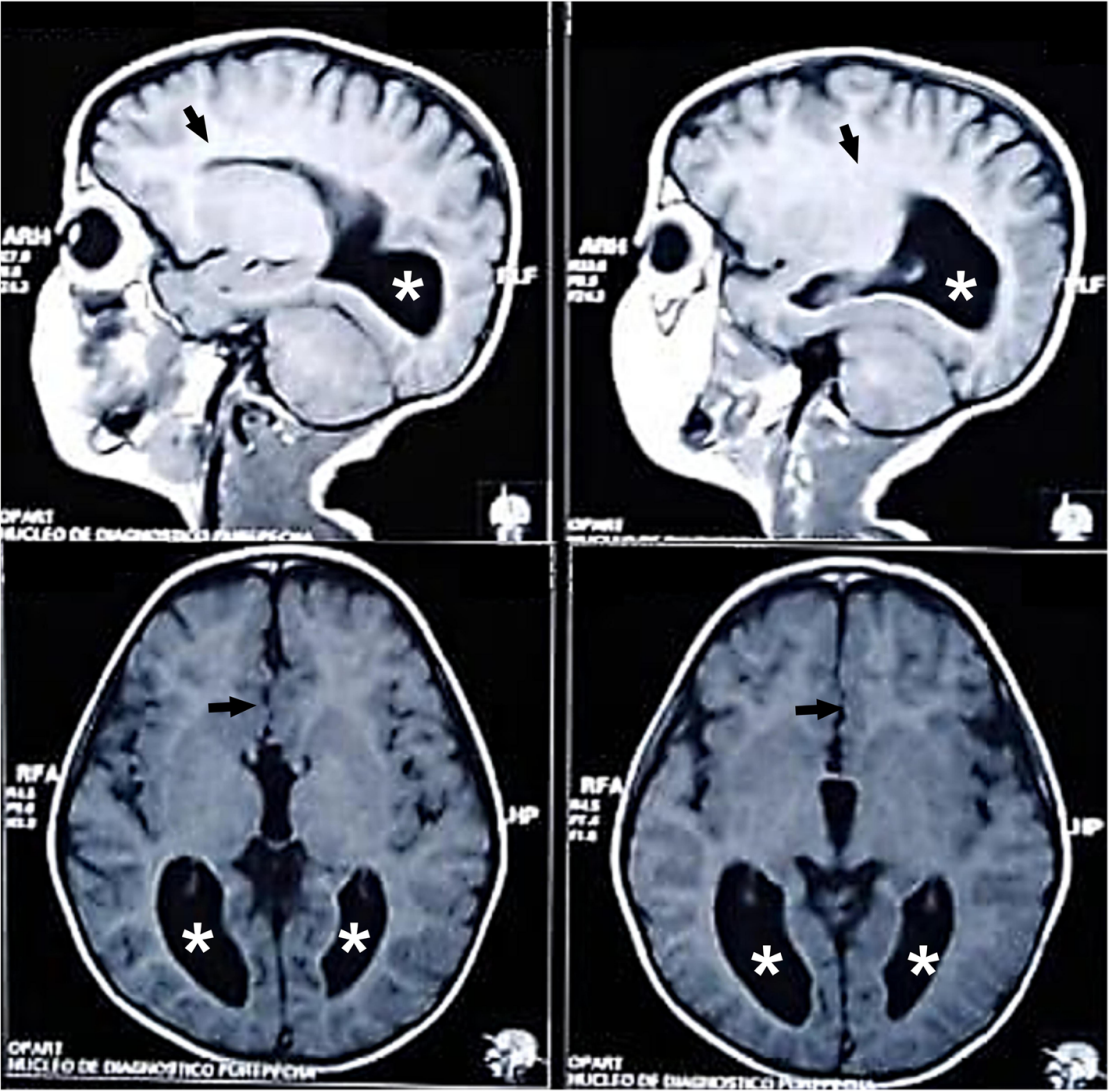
Brain magnetic resonance imaging images of the clinical case. Sagittal (*upper panels*) and transversal (*lower panels*) T1-weighted brain magnetic resonance imaging images showing total agenesis of the corpus callosum (*black arrows*) and enlargement of the occipital horns of the lateral ventricles (*white stars*).
